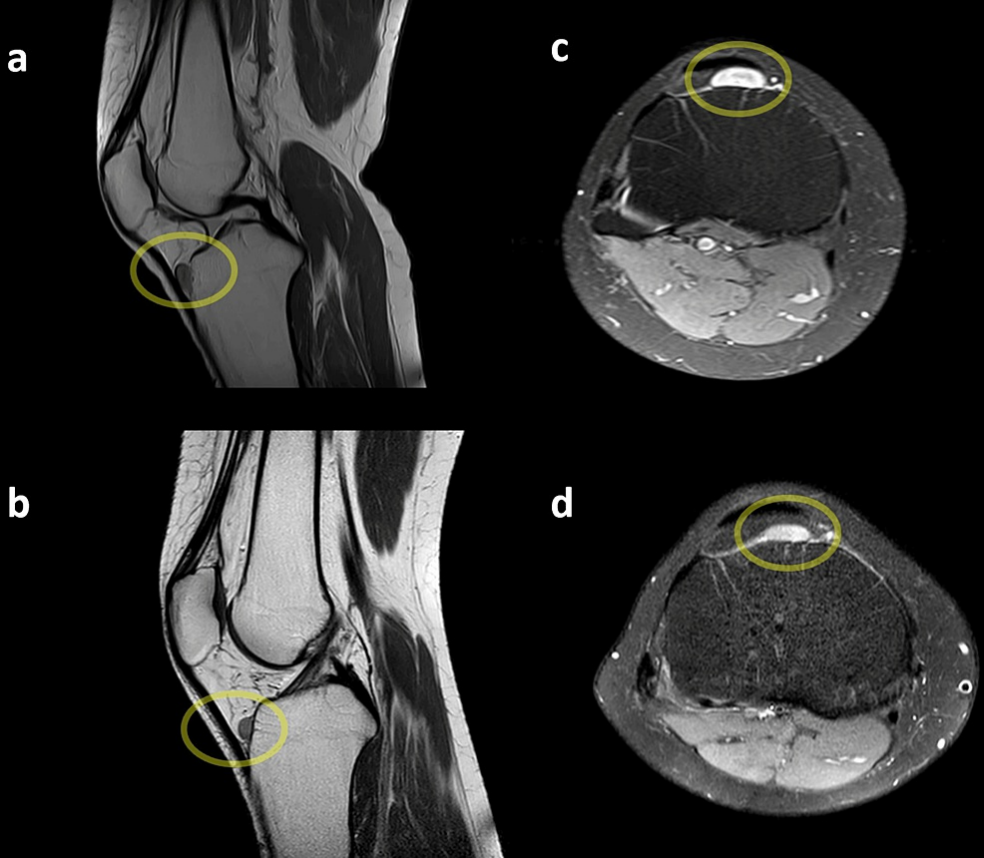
(a, c) MRI scan obtained before the patient's first surgery in 2019; (b, d) patient's current MRI scan in 2021. (a, b) In the sagittal view, T1-weighted MRI scan, the leiomyoma appears as a well-demarcated soft tissue mass with intermediate signal intensity, displaying a homogeneous nature. (c, d) In the axial view, T2-weighted MRI scan, the lesion is observed as hyperintense, indicating a brighter signal, and exhibits a well-circumscribed appearance.
